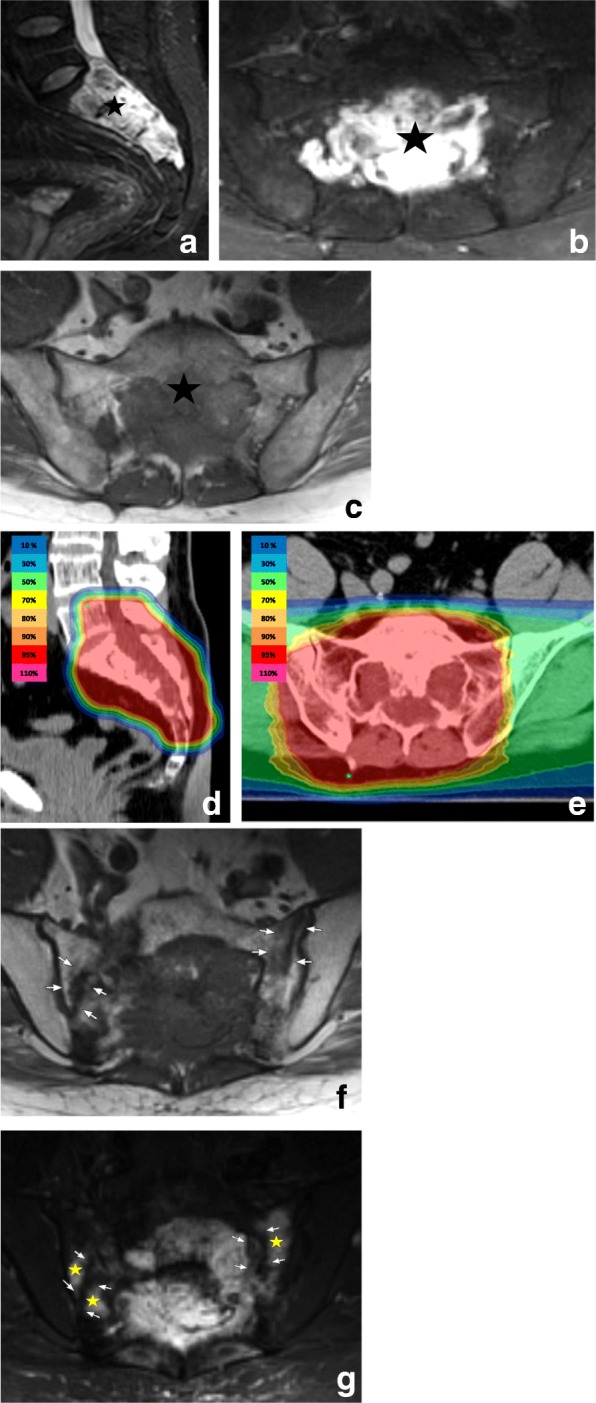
47-year-old man with an extended sacral chordoma (black star) as shown on axial T1- and T2-weighted MR-images before definitive radiotherapy (a, b, c), and on the planning CT with the corresponding dose distribution (d and e). The post-treatment MRI 12 months after radiation shows sacral insufficiency fractures in both massae laterales of the sacrum (white arrows) with corresponding hypointense linear signal abnormalities on both T1- and T2-weighted MR-images (f and g). The T2-weighted axial images (g) additionally depict the surrounding hyperintense bone marrow edema (yellow stars) which mimics tumor progression
